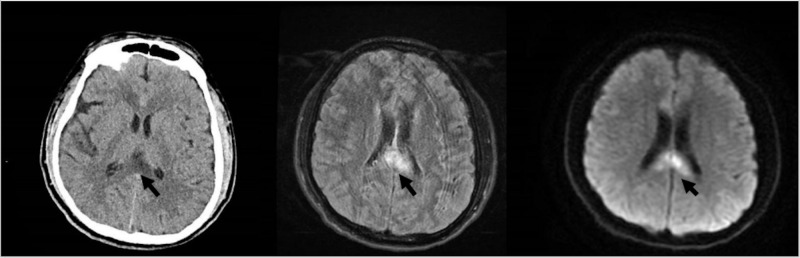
Brain imaging on first admission (A) Axial CT brain image demonstrates a nonspecific hypoattenuation in the splenium of the corpus callosum (black arrow) and questionable subarachnoid hemorrhage (not shown). (B and C) Axial MRI brain FLAIR and diffusion images demonstrate abnormal FLAIR hyperintense and diffusion restricting lesions at the splenium of the corpus callosum correlating with the CT findings (black arrows). MRI did not show any subarachnoid hemorrhage. FLAIR, fluid-attenuated inversion recovery
